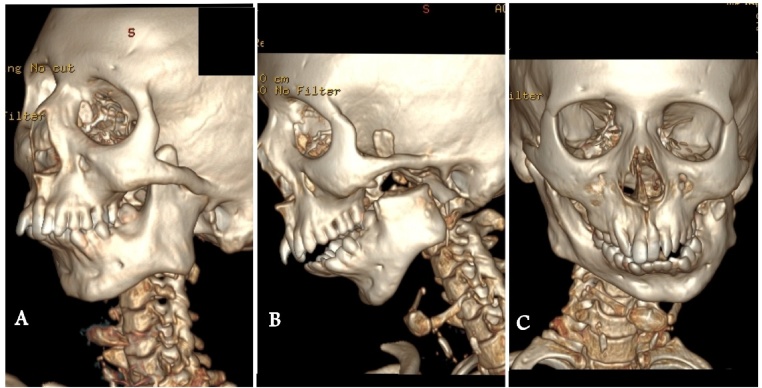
Computed Tomographic Images. A: Pre – op TMJ Ankylosis. B: Post – op TMJ Ankylosis. C: Pre – op Orthomorphic correction.
